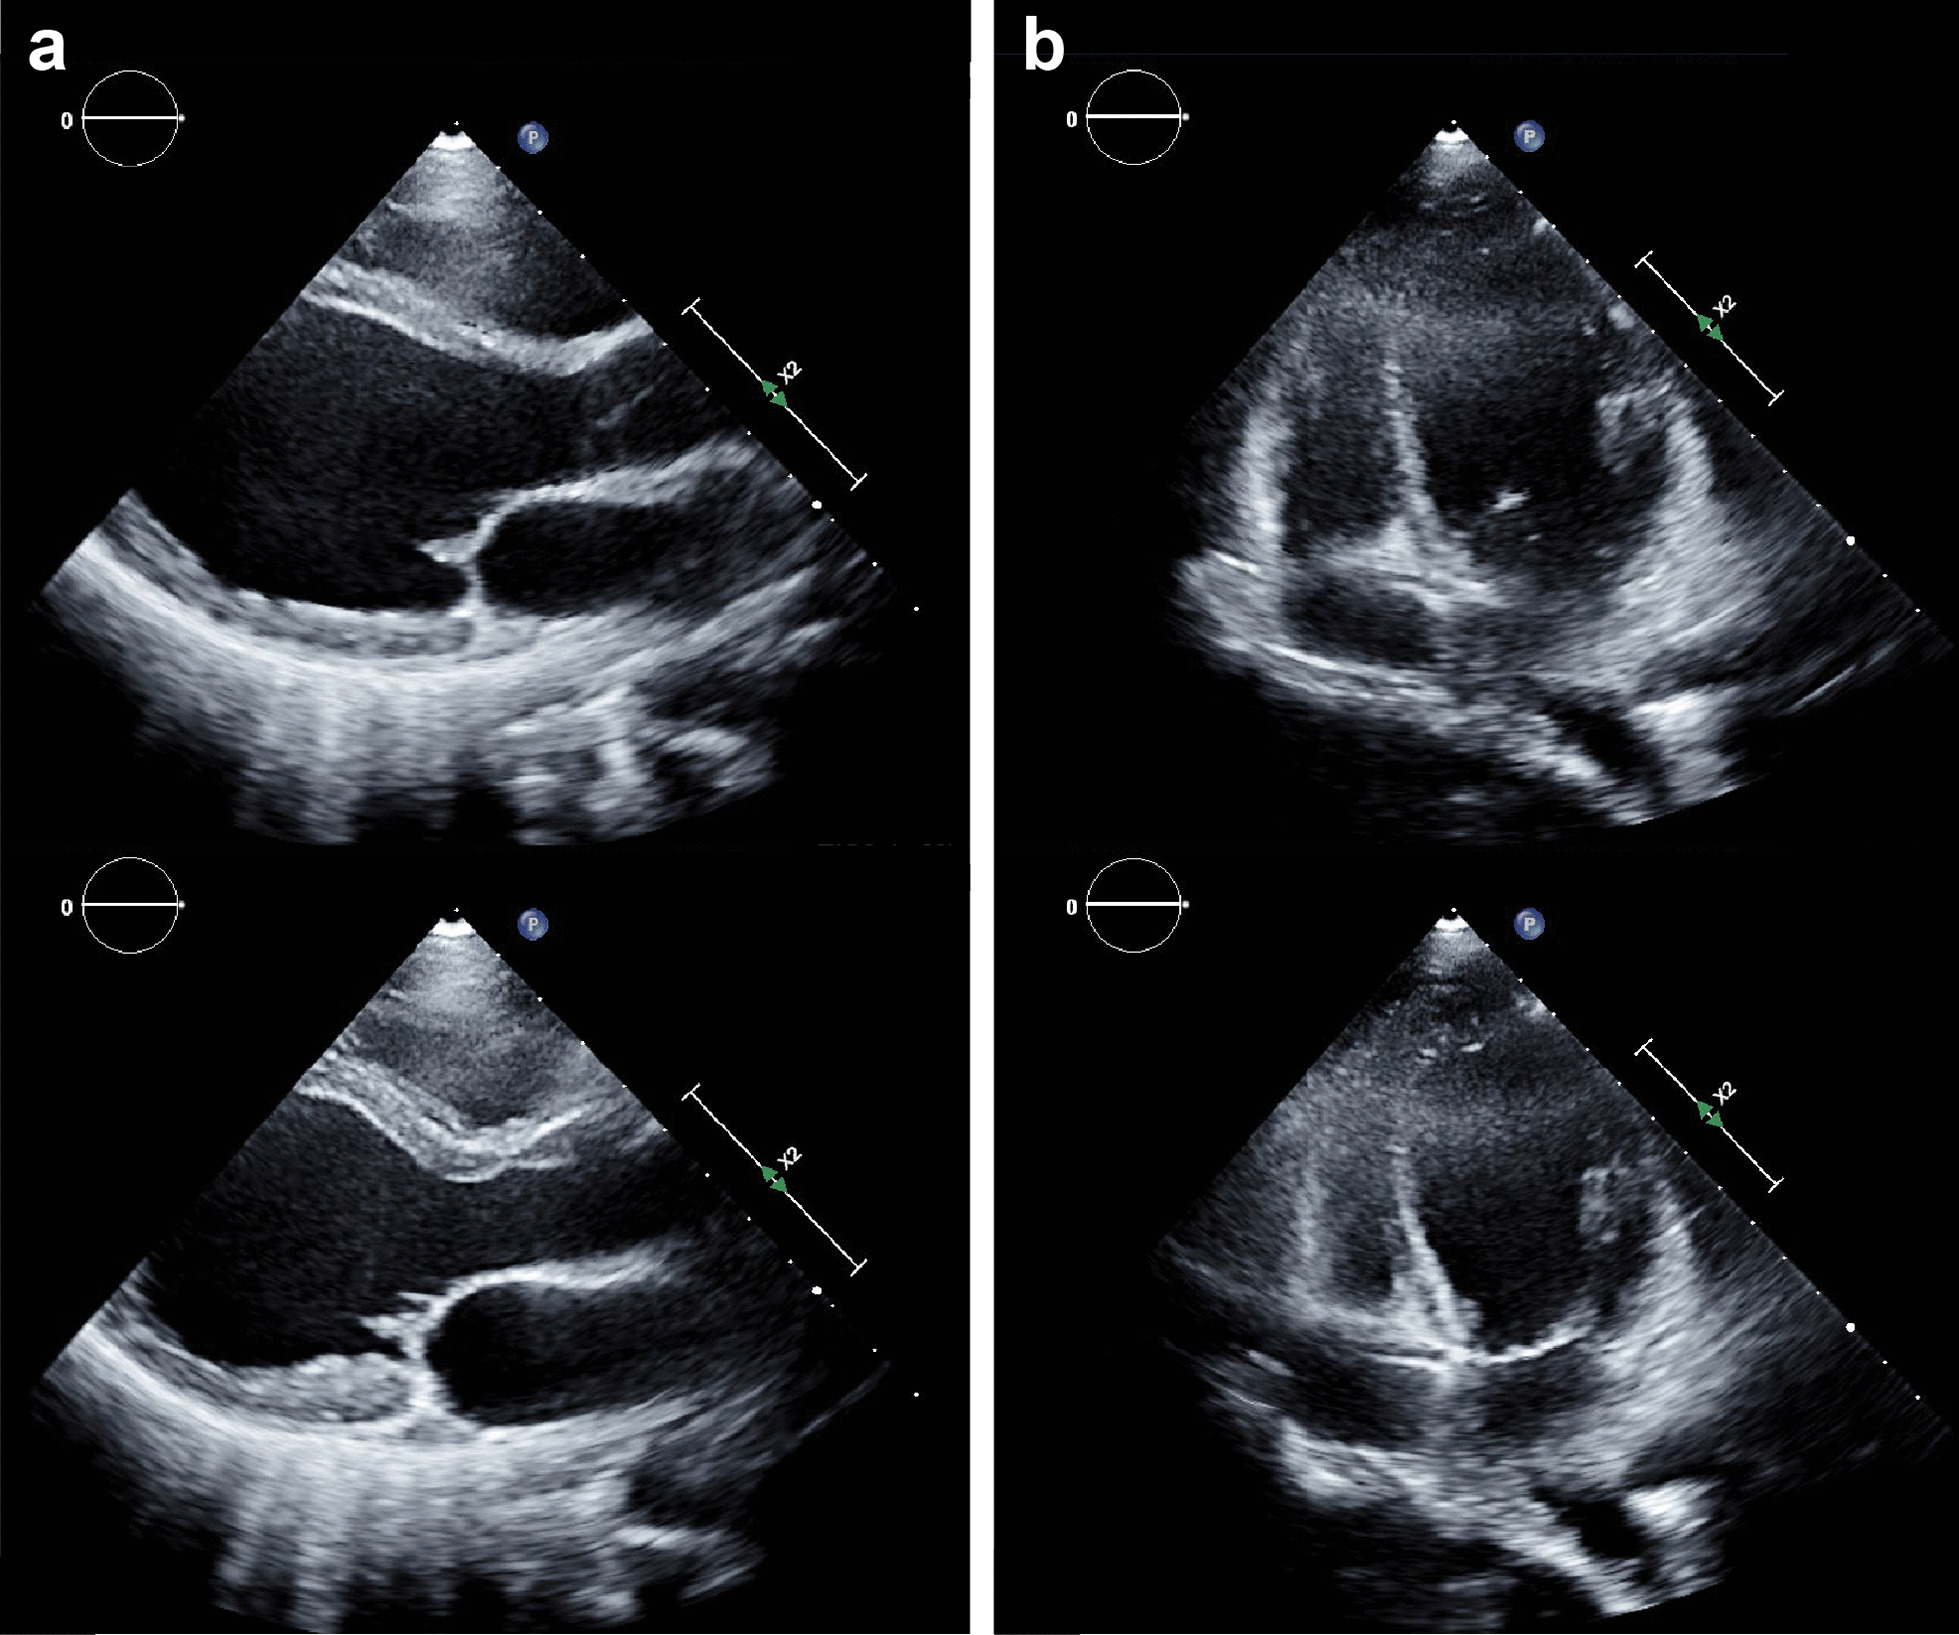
Initial transthoracic echocardiogram of end-diastolic and end-systolic phase. Mid-ventricular and apical akinesia with basal hyperkinesia are observed. a Parasternal long axis view of end-diastolic (upper) and end-systolic phase (lower). b Apical four chamber view of end-diastolic (upper) and end-systolic phase (lower)
